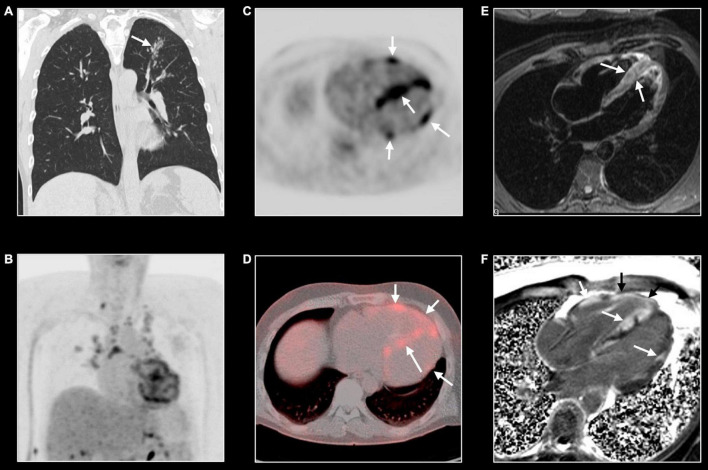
Cardiac sarcoidosis. Forty-three-year-old man known with systemic sarcoidosis admitted with recurrent ventricular tachycardia. Chest CT (A) shows enlarged mediastinal lymph nodes and perilymphatic micronodular pattern in left upper lobe (arrow), right middle and lower lobe. Fluoro-deoxy-gluclose (FDG) PET/CT shows multifocal FDG uptake in mediastinal and hilar lymph nodes (B), and strong multifocal uptake in the myocardium of left and right ventricle [arrows, (C,D)]. T2w-imaging shows myocardial edema, most pronounced in the apical half of a thickened ventricular septum [arrows, (E)] while LGE imaging shows multifocal, pronounced myocardial enhancement in left and right ventricle [arrows, (F)]. CMR and PET/CT findings are strongly suggestive of cardiac sarcoidosis with severe biventricular involvement. Cardiac biopsy showed granulomatous myocarditis. Because of the cardiac arrhythmias, the patient received an ICD implantation with several appropriate shocks. Unfortunately, the patient evolved toward biventricular heart failure for which he underwent a heart transplantation. Eight years later, the clinical history is uneventful.
